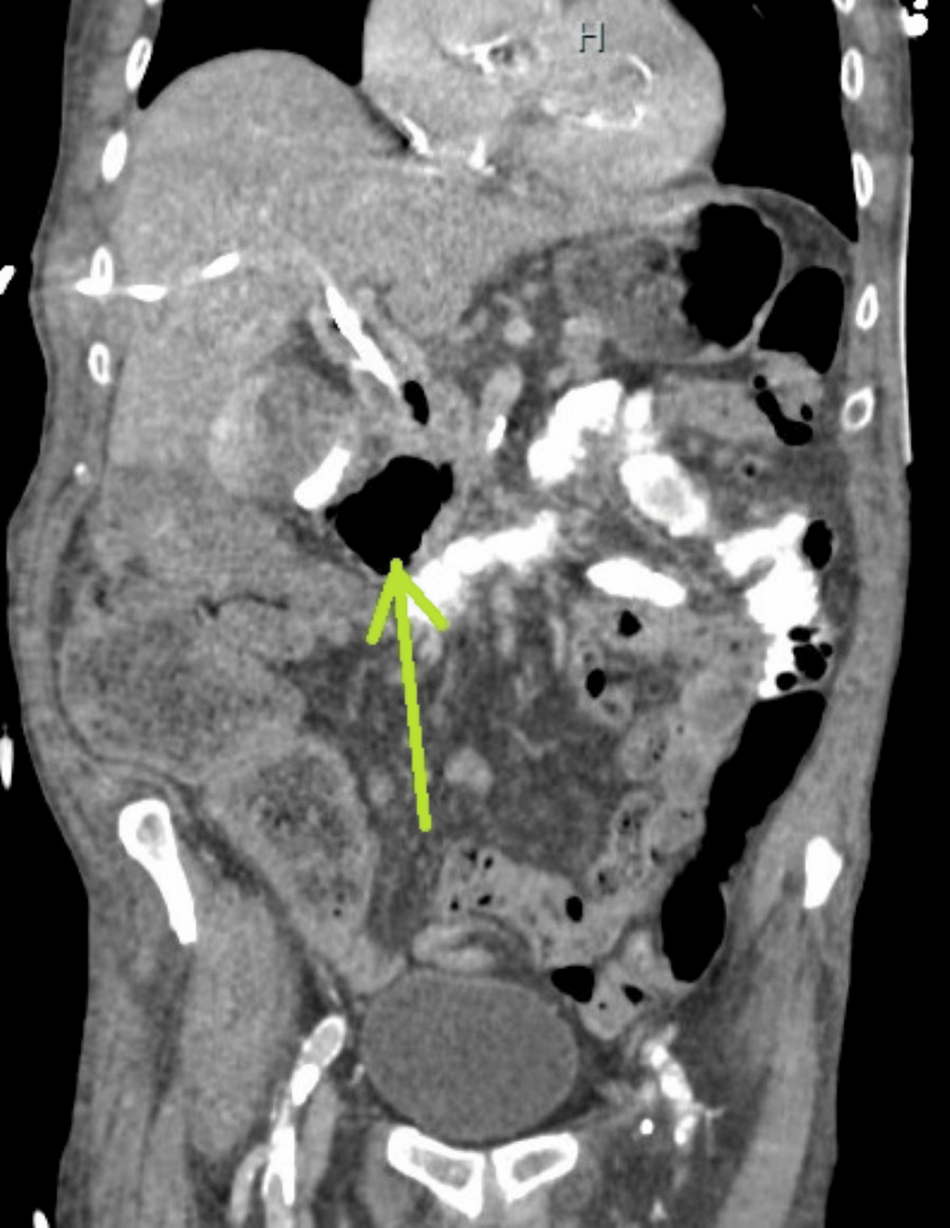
Another view of coronal contrast-enhanced CT of the abdomen showing a periampullary duodenal diverticulum compressing the distal common bile duct.
